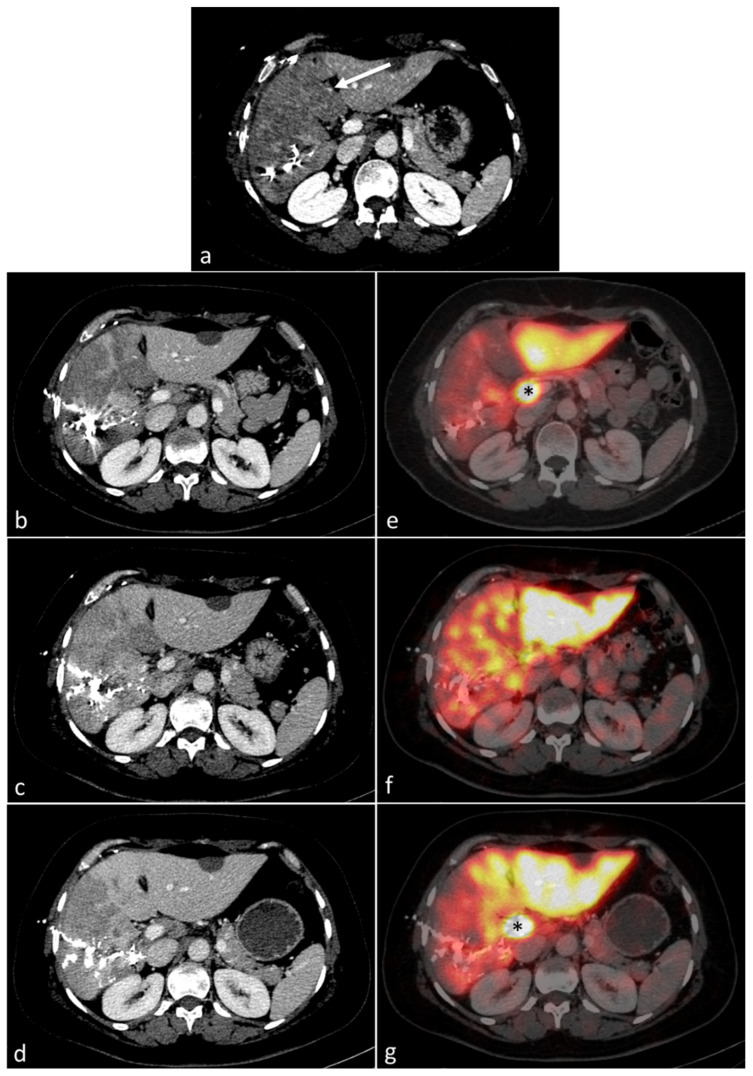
Axial portal venous phase CT at day 1 after eLVD (a) showing hypoattenuation in S4 (arrow), which progressively recovers at days 7 (b), 14 (c) and 21 (d). Corresponding 99mTc-mebrofenin SPECT-CT images of the same patient showing progressive increase in S4 function at days 7 (e), 14 (f) and 21 (g). Please note artifactual activity due to the radiotracer accumulation in the main bile duct (black asterisk).
